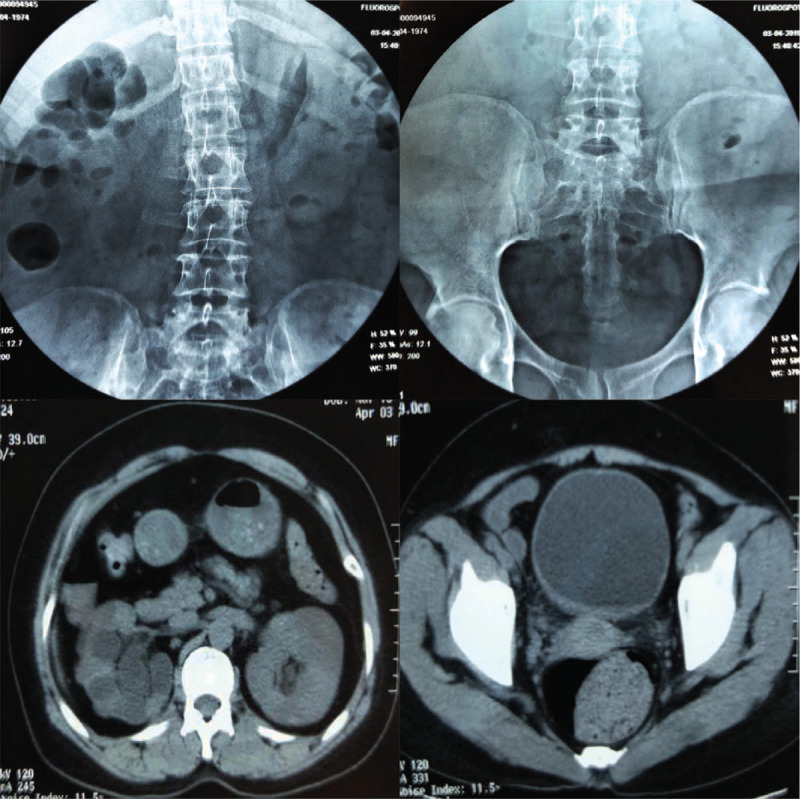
No residual kidney and bladder stone was detected on postoperative KUB x-ray and CT scans. CT = computed tomography, KUB = kidney ureter bladder.
